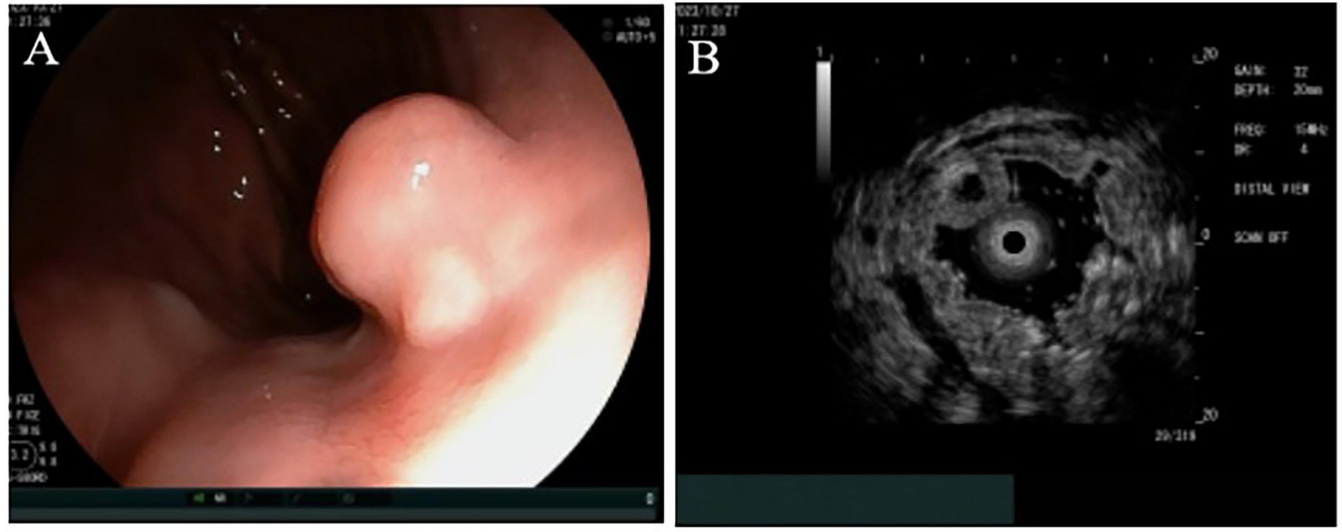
Endoscopic findings. (A) A hemispherical smooth submucosal mass was observed on white light endoscopy. (B) Endoscopic ultrasonography showed a hypoechoic mass with uniform internal echo originating from the third (submucosal) layer.
